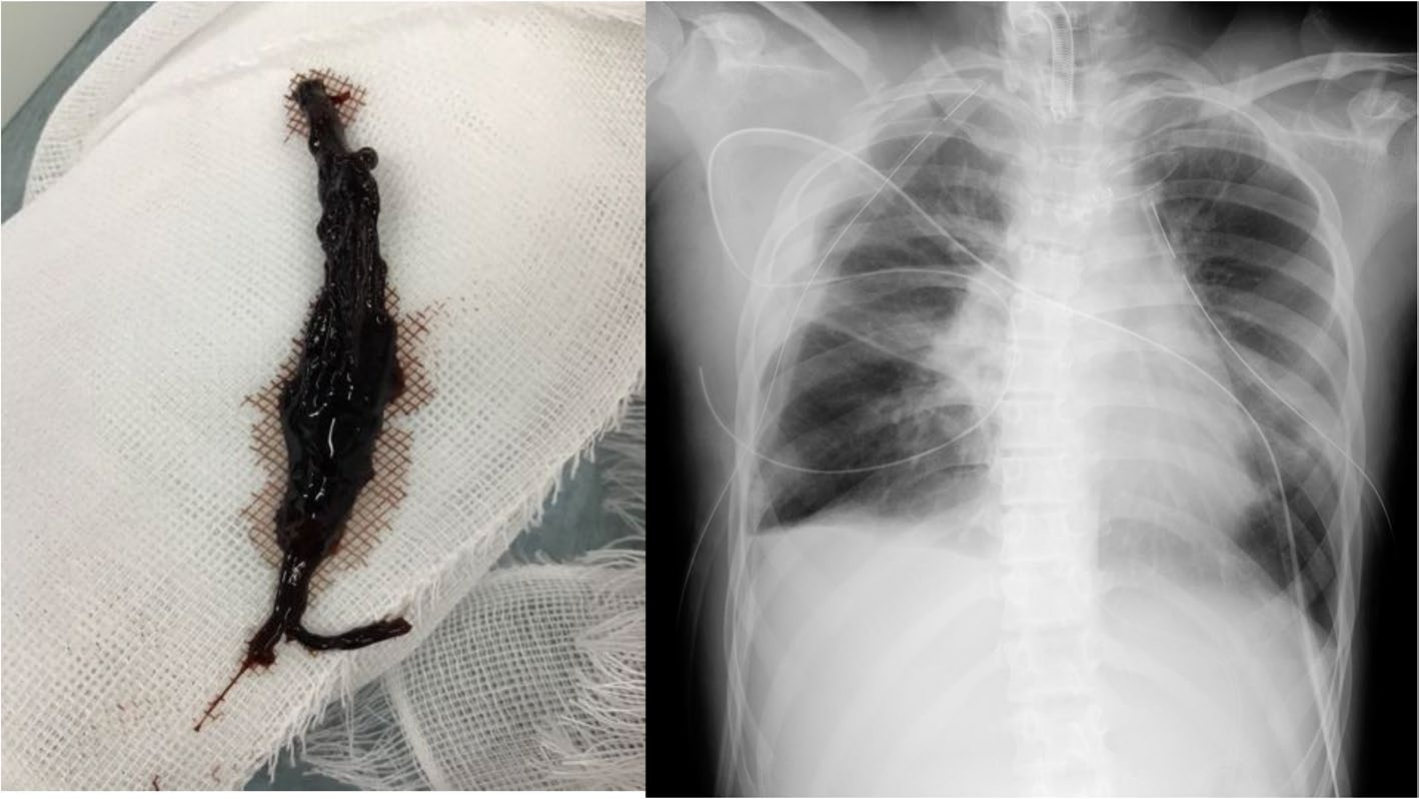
Organized blood clot removal from tracheostomy site and radiograph after veno-venous extracorporeal membrane oxygenation withdrawal. The organized large clot was removed on day 9 (left), and apparent atelectatic was not observed in the left lung field after weaning from veno-venous extracorporeal membrane oxygenation on day 10 (right)
